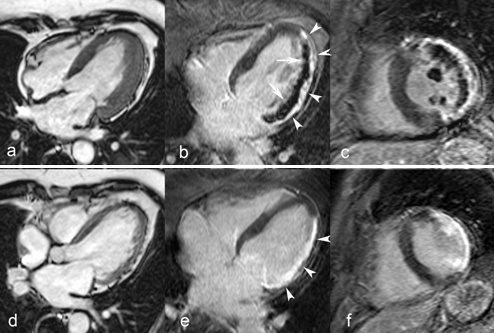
Fig. 8Extensive lateral MI in 68 year–old man studied 1 week and 4 months after the acute event. Proximal Cx coronary artery occlusion treated by primary PCI but complicated by no reflow. Cine MRI in horizontal long–axis shows thickened LV lateral wall due to myocardial edema and inflammation in the acute phase (a), followed by marked wall thinning at 4 months (d). Horizontal long–axis and midventricular short–axis late ce–MRI show an extensive subendocardial no reflow zone at 1 week (*arrows*
